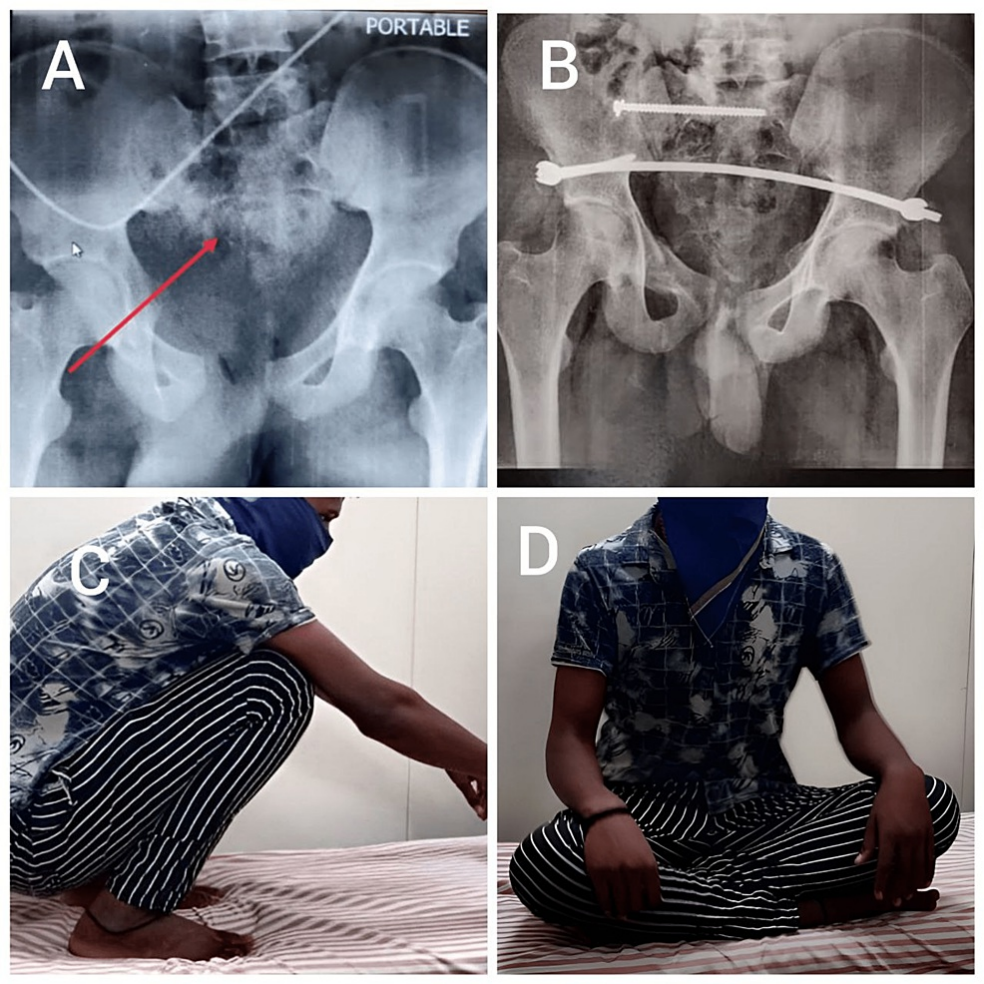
A) A 32-year-old male with anterior and posterior pelvic ring injury. B) Postoperative X-ray of the same patient. C and D) Range of motion at six months.
